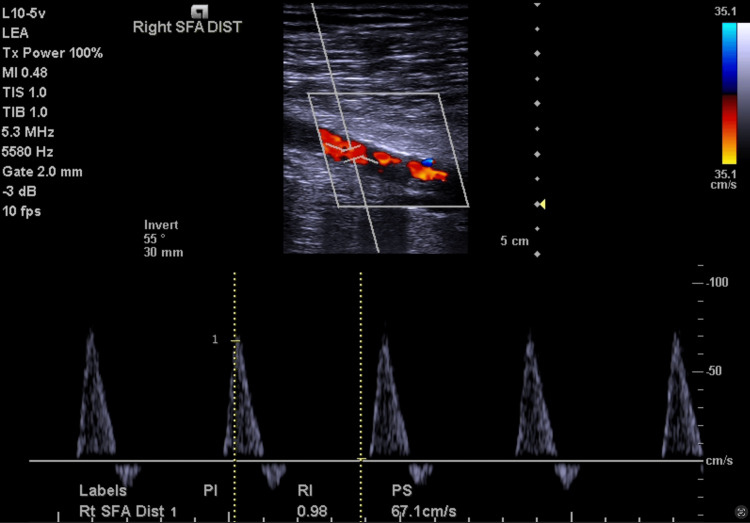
Arterial ultrasound revealed biphasic waveforms on the right side with moderate infrapopliteal disease and decreased velocity
